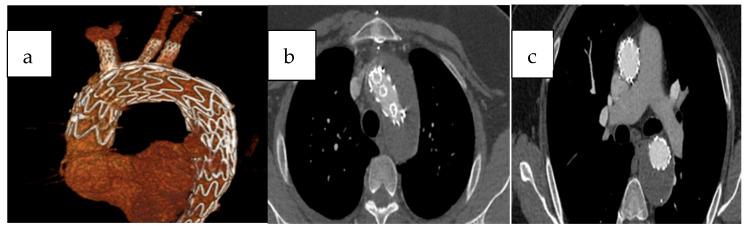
A contrast-enhanced computed tomography (CT) image obtained 6 months after the procedure with volume-rendered 3D reconstruction (a) The original axial image clearly demonstrates the contrast enhancement of the stents in the triple arch branches (b) and the thrombosed false lumen in the descending aorta (c).
